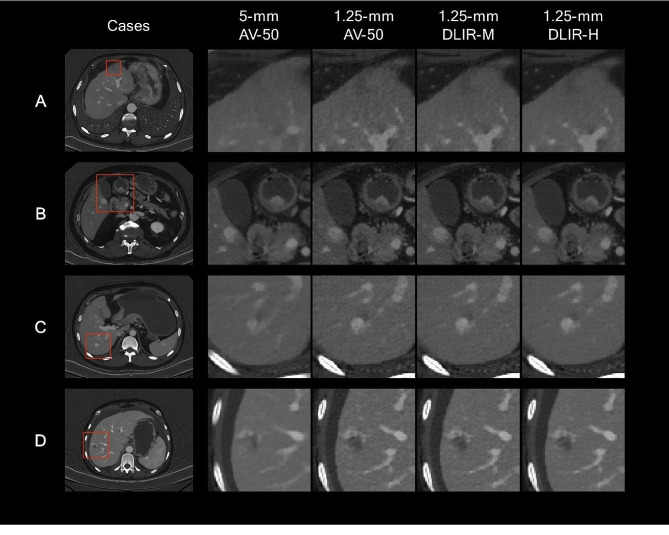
Four examples of abdominal CT studies. These portal-venous phase abdominal CT studies were reconstructed into iodine maps using 5-mm AV-50, 1.25-mm AV-50, 1.25-mm DLIR-M, and 1.25-mm DLIR-H algorithms; and present with the same windowing (width of 15.0 mgI/mL, level of 5.0 mgI/mL). (A) A patient underwent CT scan for cancer staging. A low-density hepatic lesion was better visualized in 1.25-mm images than the 5-mm AV-50 image. The 1.25-mm DLIR image better balanced the image noise and spatial resolution. (B) A patient underwent CT scan for cancer staging. The enhanced hepatic lesion can be detected in all the images, while the details of the texture and boundary were better visualized in 1.25-mm images. The lesion of the stomach can be detected in all the images, but the enhanced range was better displayed with lower image noise in 1.25-mm DLIR-H images, potentially allowed more accurate staging. (C) A patient underwent CT scan for hepatic lesion. Compared with the 5-mm AV-50 image, the 1.25-mm images better showed the enhance pattern of the lesion. The 1.25-mm DLIR-H images presented the lesion with more details and lower image noise. (D) A patient underwent CT scan for hepatic lesion. A hepatic lesion with surrounding enhancement was detected. The lesion texture of is better depicted with 1.25-mm images. The 1.25-mm DLIR-H images best balanced the image noise, and spatial resolution
